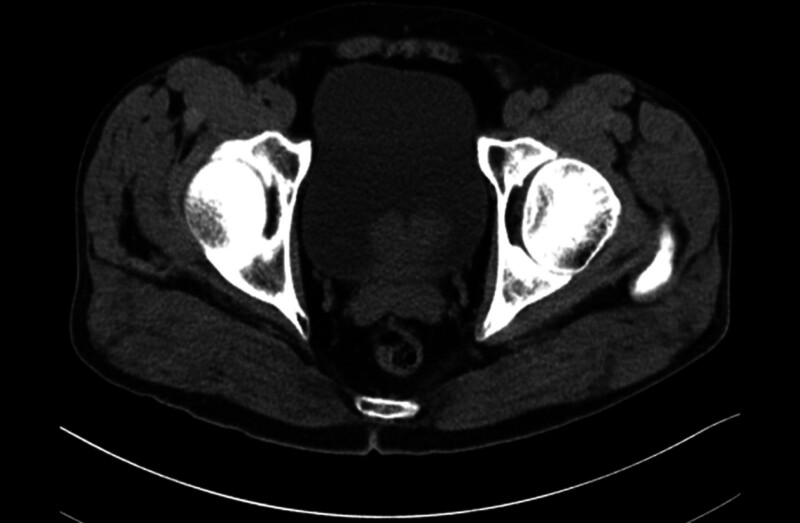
Pelvic CT: an irregular, mass-like high-density shadow is seen in the posterior bladder wall, poorly demarcated from the prostate.
